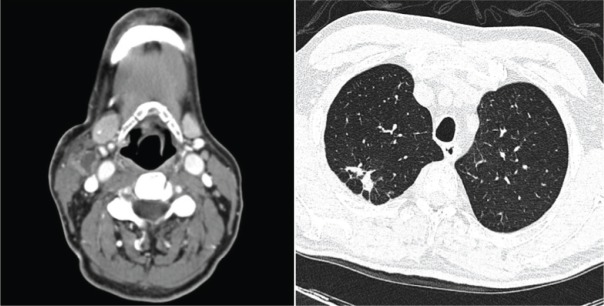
(A) Contrast enhanced CT on day +70 after HSCT presented right cervical lymph nodes enlargement with central necrosis. (B) Highresolution chest CT revealed old pulmonary scar lesions with pleural thickening in the right upper lobe.
